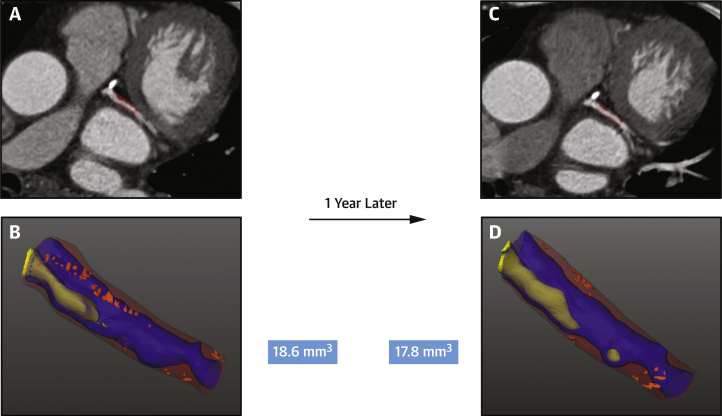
Low-Attenuation Plaque Progression on CCTA in a Patient With Low Lp(a) Coronary computed tomography angiography (CCTA) of a patient with low serum lipoprotein(a) [Lp(a)] concentration (9.2 mg/dL) showing atherosclerotic plaque in the left circumflex artery (A and C) with evidence of mixed plaque on automated plaque assessment (red overlay). Low-attenuation plaque is visualized in bright orange on the 3-dimensional reconstruction (B and D) and does not appear to progress on serial scanning (baseline volume 18.6 mm^3^, 1-year volume 17.8 mm^3^).
